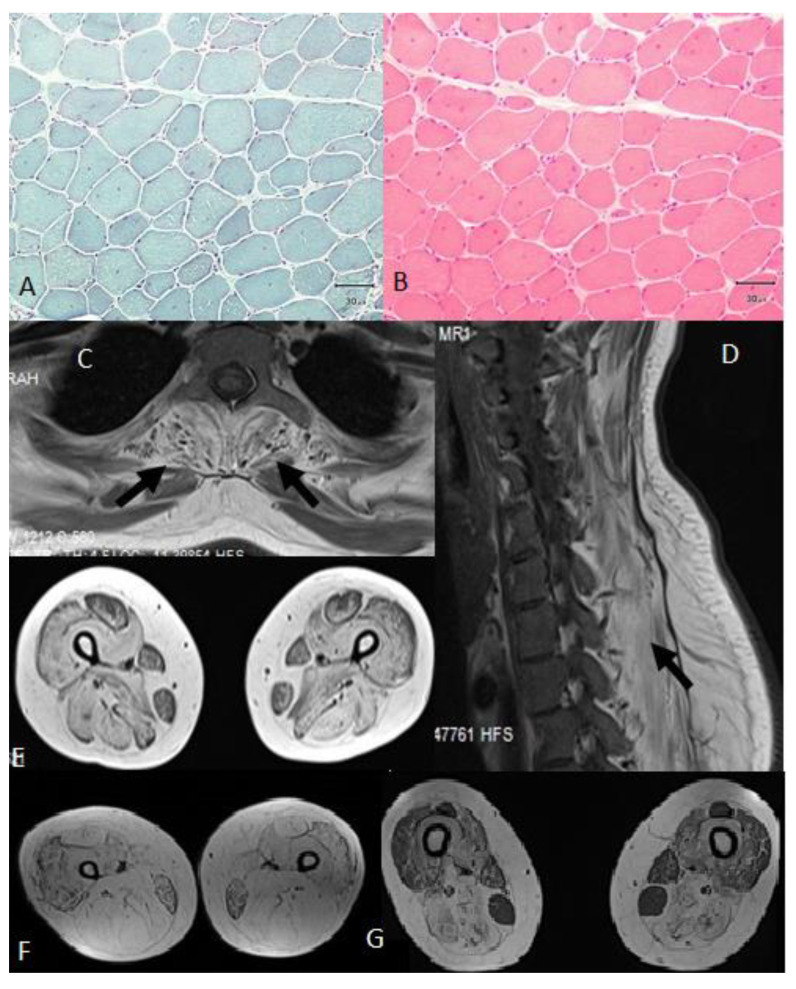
(A) and (B) A muscle biopsy from the deltoid muscle of patient D showed myopathic changes with increased numbers of internal nuclei (over 10%) and with a few angular atrophic fibers; (C), (D) and (E) A muscle MRI of patient D showed paraspinal muscle involvement and good preservation of the sartorius and gracilis muscles in the lower limbs. (F) The muscle MRI of patient A showed advanced fatty transformation, but fairly good preservation of the gracilis muscles. (G) The muscle MRI of patient B showed clear pathology of the adductor magnus, adductor longus, biceps femoris, semitendinosus, and semimembranosus muscles and sparing of the gracilis muscles with good preservation of the rectus femoris and sartorius muscles.
